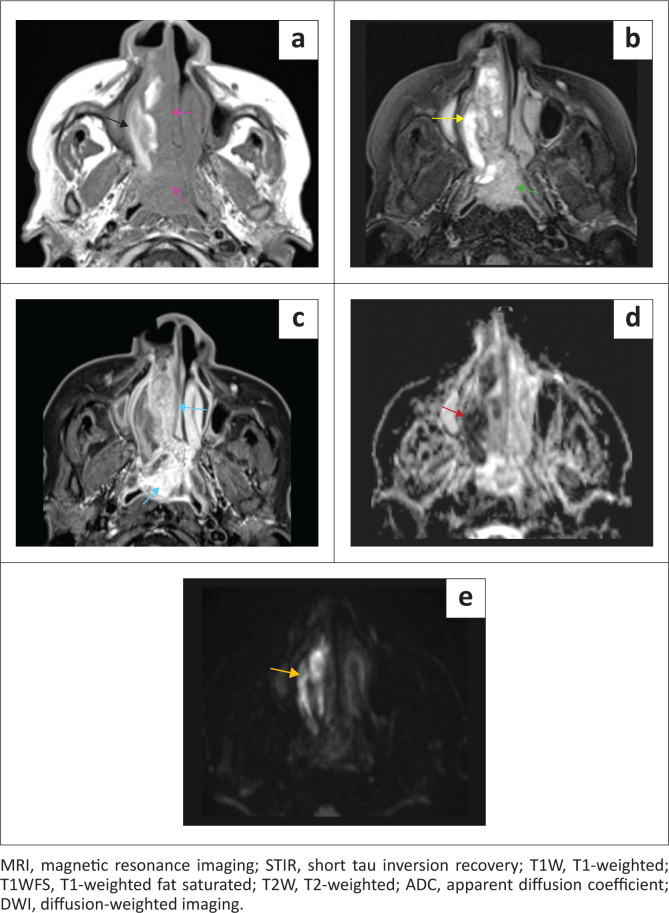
Multisequence axial MRI images (a) T1W, (b) STIR, (c) T1WFS post contrast, (d) ADC, and (e) DWI. (a) T1W isointense (purple arrows) with a hyperintense component (black arrow). (b) T2W hyperintense (green arrow) with a markedly hyperintense component (yellow arrow). (c) Heterogeneous post-contrast enhancement (blue arrows). (d, e) T1W hyperintense component demonstrates ADC hypointensity (red arrow) and DWI hyperintensity (orange arrow).
